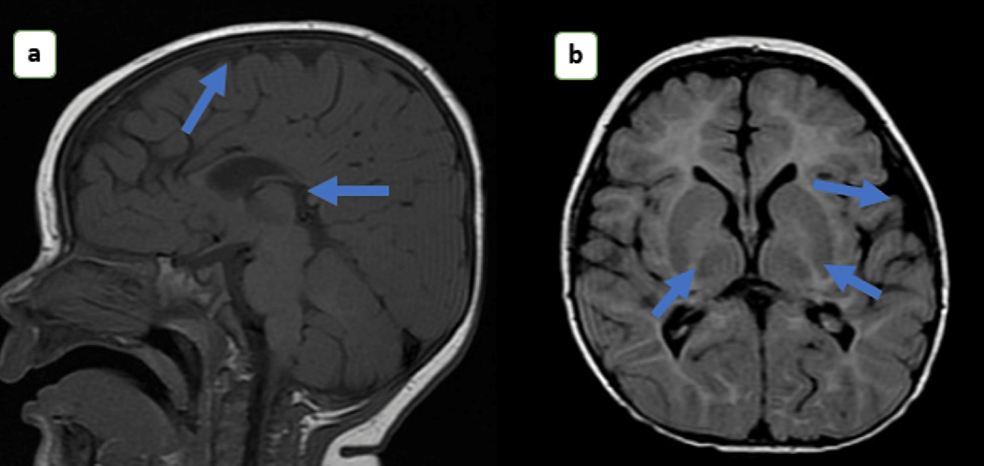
MRI brain showing decreased white matter volume in the bilateral parietal region, right occipital region, diffuse cerebral atrophy, diffuse thinning of the corpus callosum, and minimal myelination of internal capsule, periventricular white matter suggestive leukodystrophy (a, b).
